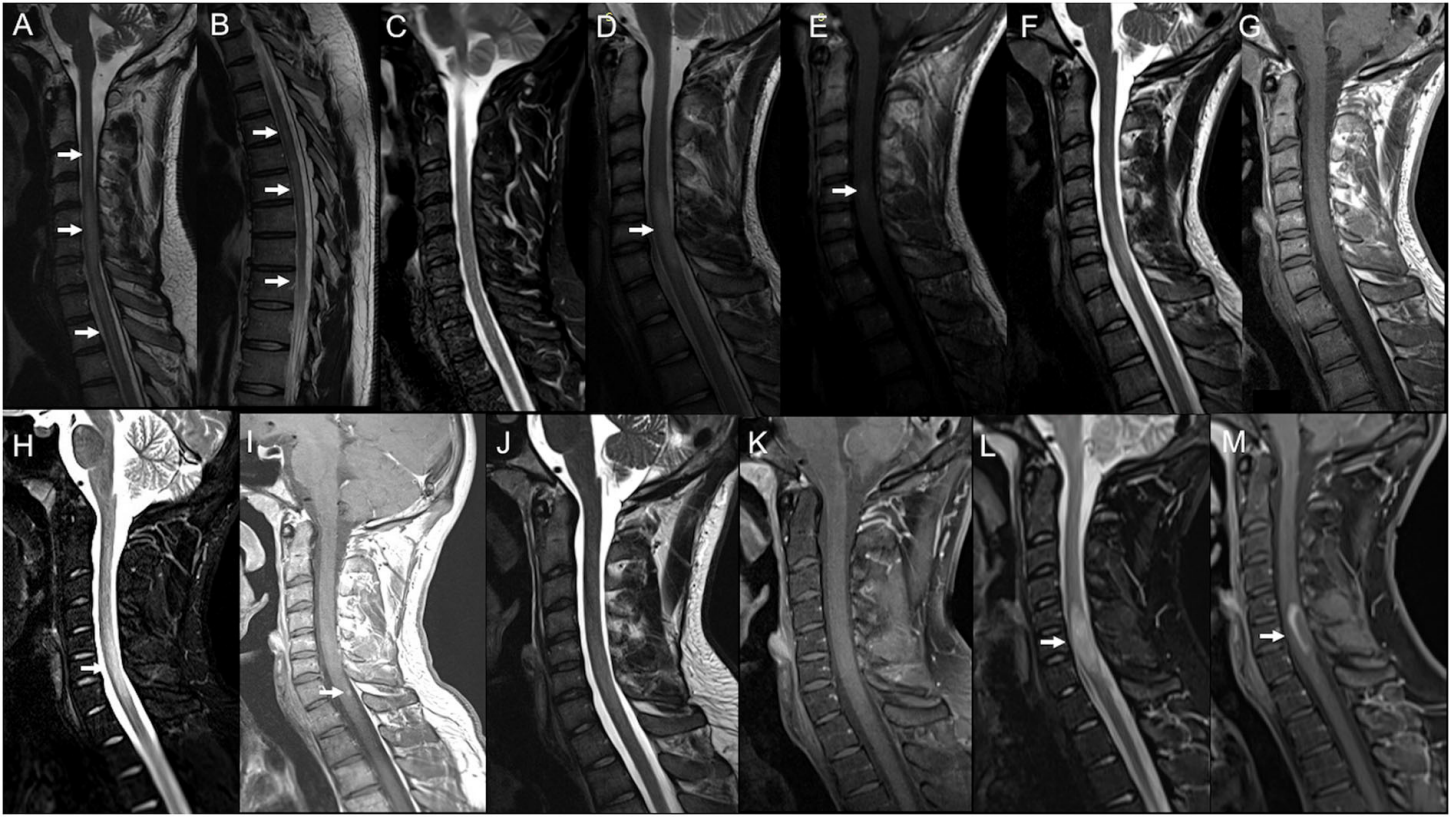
Serial spinal cord MRI scans in Case 1 (A–C) and Case 2 (D–M). Case 1: Cervical (A) and thoracic (B) spinal cord MRI obtained 10 days after Ad26.COV2.S first dose showed a T2-weighted hyperintensity irregularly extending from C3 to medullaris conus with swelling from C4 to C7 (arrows) and no gadolinium enhancement (not shown). (C) 8-months MRI follow-up showing the complete resolution of the swelling and marked amelioration of the signal abnormality, barely visible on Short Tau Inversion Recovery (STIR) images. Case 2: (D) Spinal cord MRI performed 3 months after Tdap and IVP vaccine administration, showing a T2-weighted hyperintensity from C5 to C7 (arrow) with swelling and blurred contrast-enhancement on T1-weighted sequence (E) (arrow). (F) 6-months MRI control showing the cervical lesion shrinkage on T2-weighted sequences and no more contrast-enhancement (G). (H) Spinal cord MRI obtained 8 days after the BNT162b2 second dose, showing swelling on STIR (arrow) and gadolinium enhancement [(I); arrow] of the previously detected lesion. (J) 4-months MRI showed showing marked reduction of the signal abnormality and no more enhancement on the T1-weighted image (K). (L) Spinal cord MRI obtained 62 days after the BNT162b2 third dose, showing a new swelling (arrow) and gadolinium ring enhancement (M) of the known cervical lesion.
